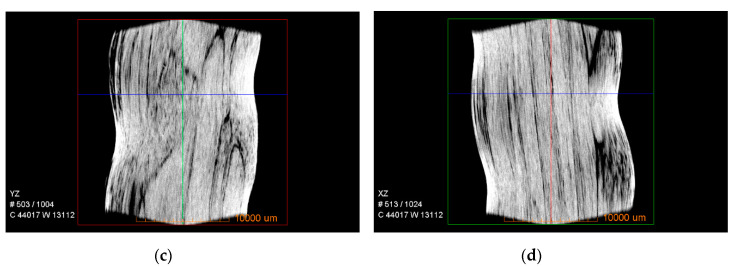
3D CT images of the internal structure of GFRP bars of: (a) YZ-plane; (b) XY-plane; (c) YZ-plane; and (d) XZ-plane.
